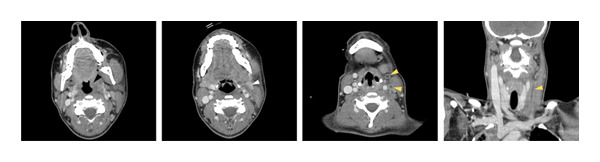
CT‐scan (axial and coronal view) in which enlargement of the left palatine tonsil (black arrow), left internal jugular vein thrombosis (yellow arrows), and left cervical lymph node (white arrow) can be seen.
